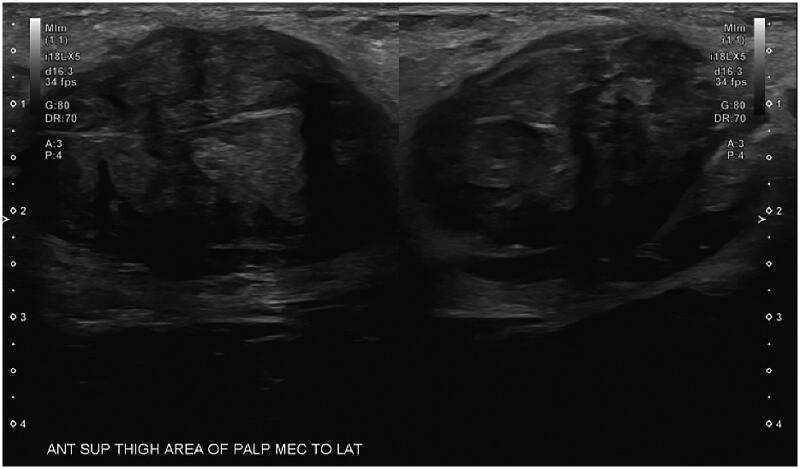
Ultrasound of the left upper thigh demonstrating a hypoechoic mass measuring 3.5 x 3 x 2.4 cm.
